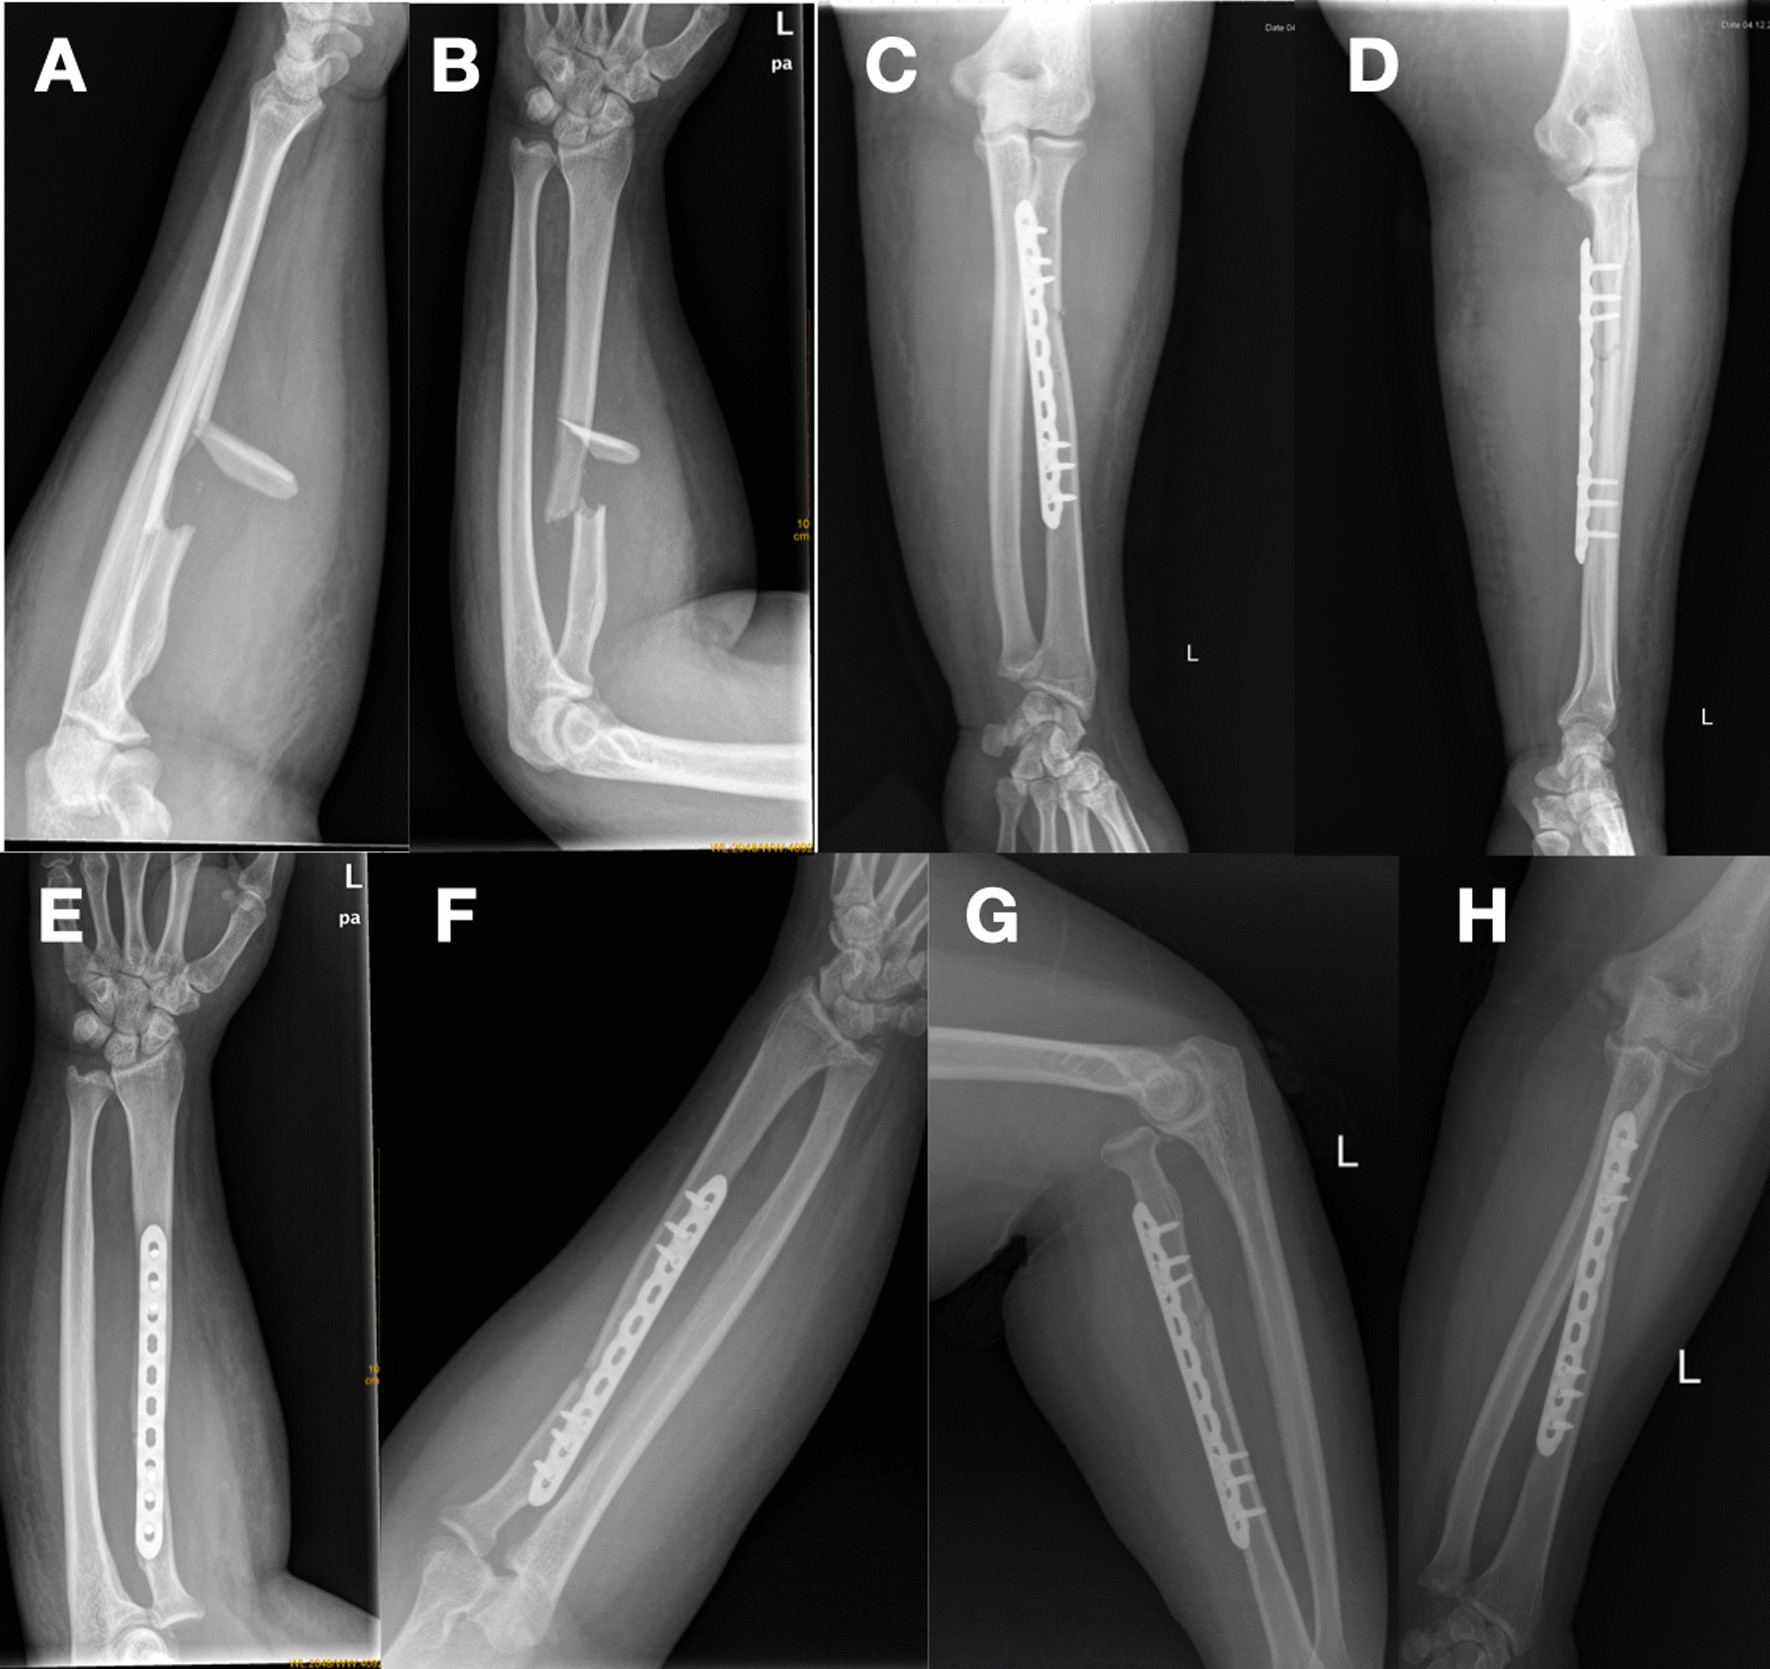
A, B Preoperative radiographs revealed a comminuted fracture of the proximal third of the radius; C, D The immediate postoperative radiograph; E, F 4th week after operation. G, H 12 months after the operation
